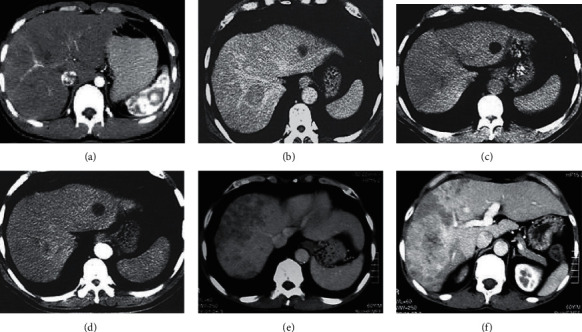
CT imaging results of acute liver injury caused by sepsis. (a–f) The CT images of patients with normal liver, grade I, grade II, grade III, grade IV, and grade V, respectively.
